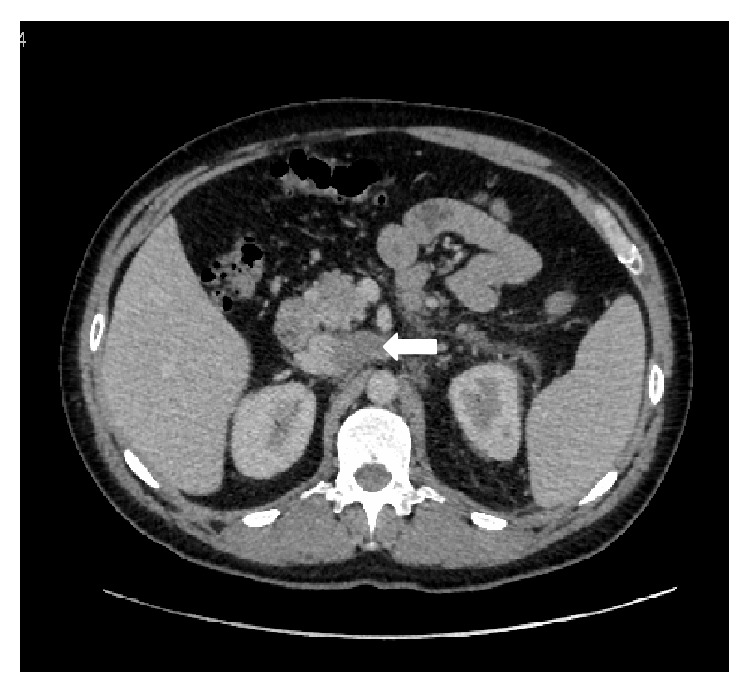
Computerized tomography (CT) scan of the abdomen demonstrating the thrombus extended from the left renal vein to the IVC. The arrow indicates the 40 mm thrombus.
